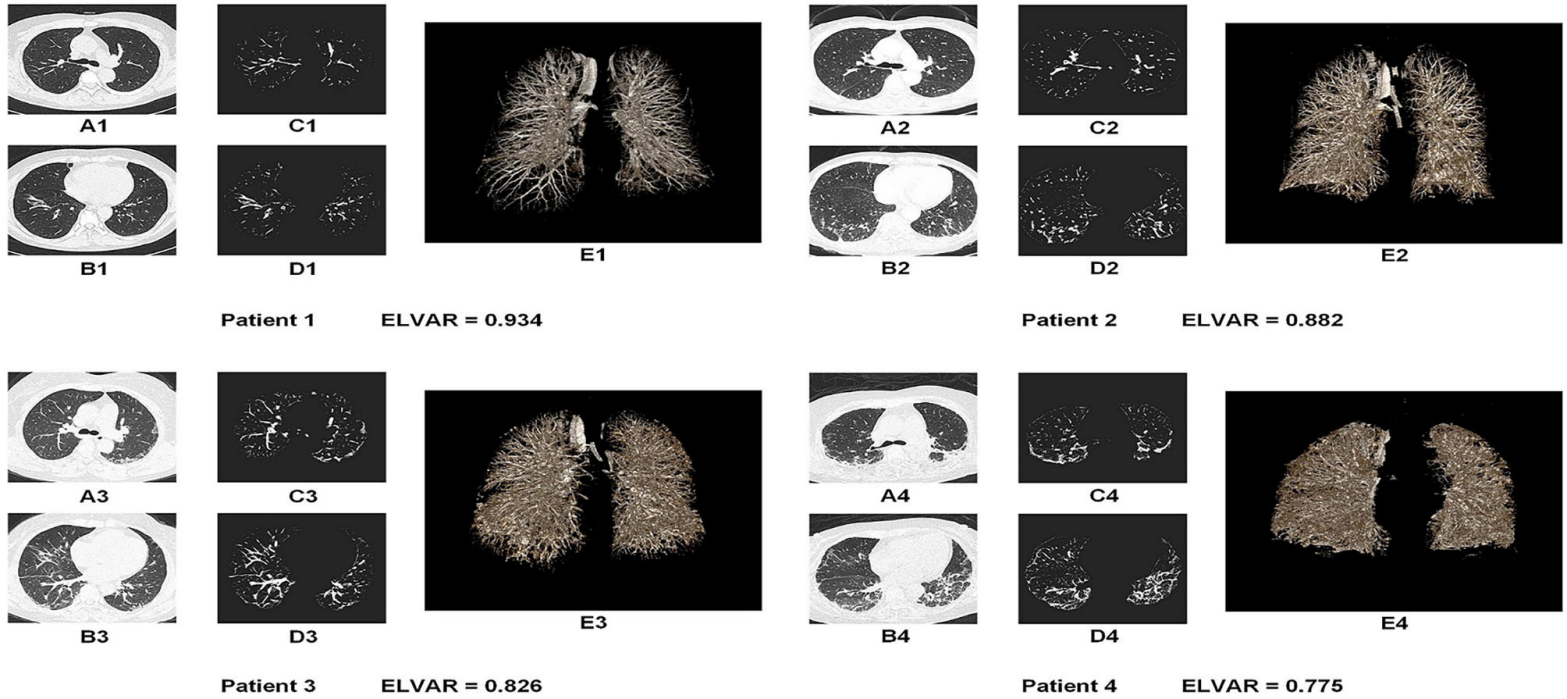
Lung images of four MDA5^+^DM patients with different value of ELVAR. (A,B) Original HRCT images in the different level of lung tissue (A was the section from bifurcation of the trachea and B was the section above the top of diaphragm); (C,D) The lung interstitial enhancement images from A and B based on computer aided analysis technique; (E) High precision three dimensional images of the lung interstitium from the same person.
